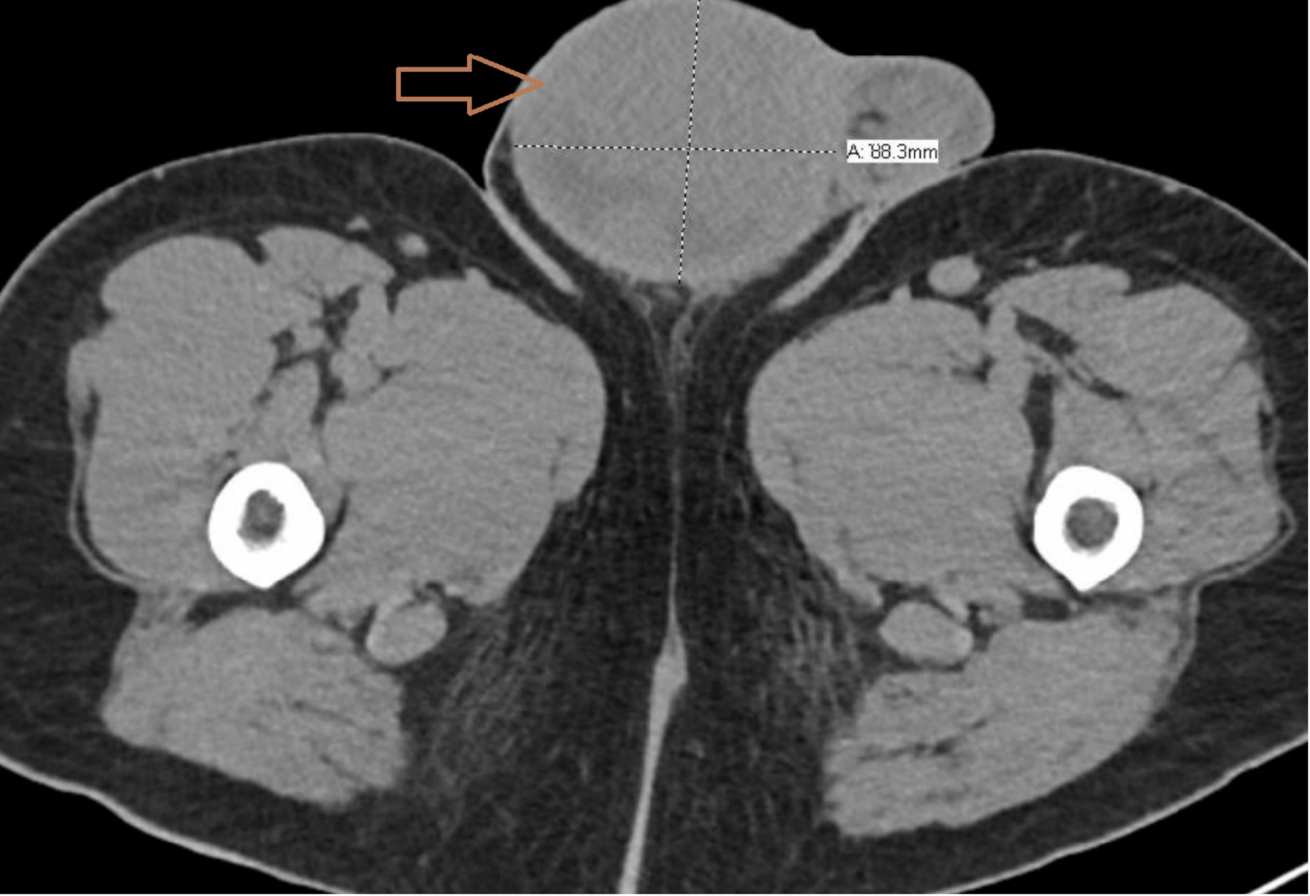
Right testicular solid mass of 8.1 cm x 8.1 cm size seen on CT scan (arrow) CT, computed tomography
